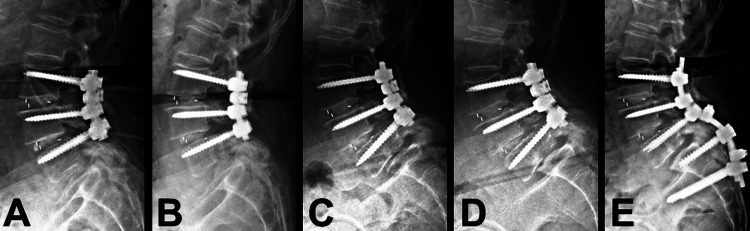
Series of radiographs during subsequent follow-ups after L3-5 TLIF The series of radiographs demonstrate the different time points of follow-up after L3-5 TLIF (A: immediate postoperative; B: two months postoperative; C: three months postoperative; and D: six months postoperative). The patient experienced a fall two months after TLIF. The radiograph arranged immediately after the episode (B) revealed no significant change, compared with the postoperative status. Elongated anterior-posterior length over L5 body with progressive listhesis was observed at postoperative three- and six-month follow-ups (C and D respectively). After the secondary surgery, L5 total laminectomy and extended fixation from L3 to S2 were performed to restore lumbosacral alignment (E) TLIF: transforaminal lumbar interbody fusion
